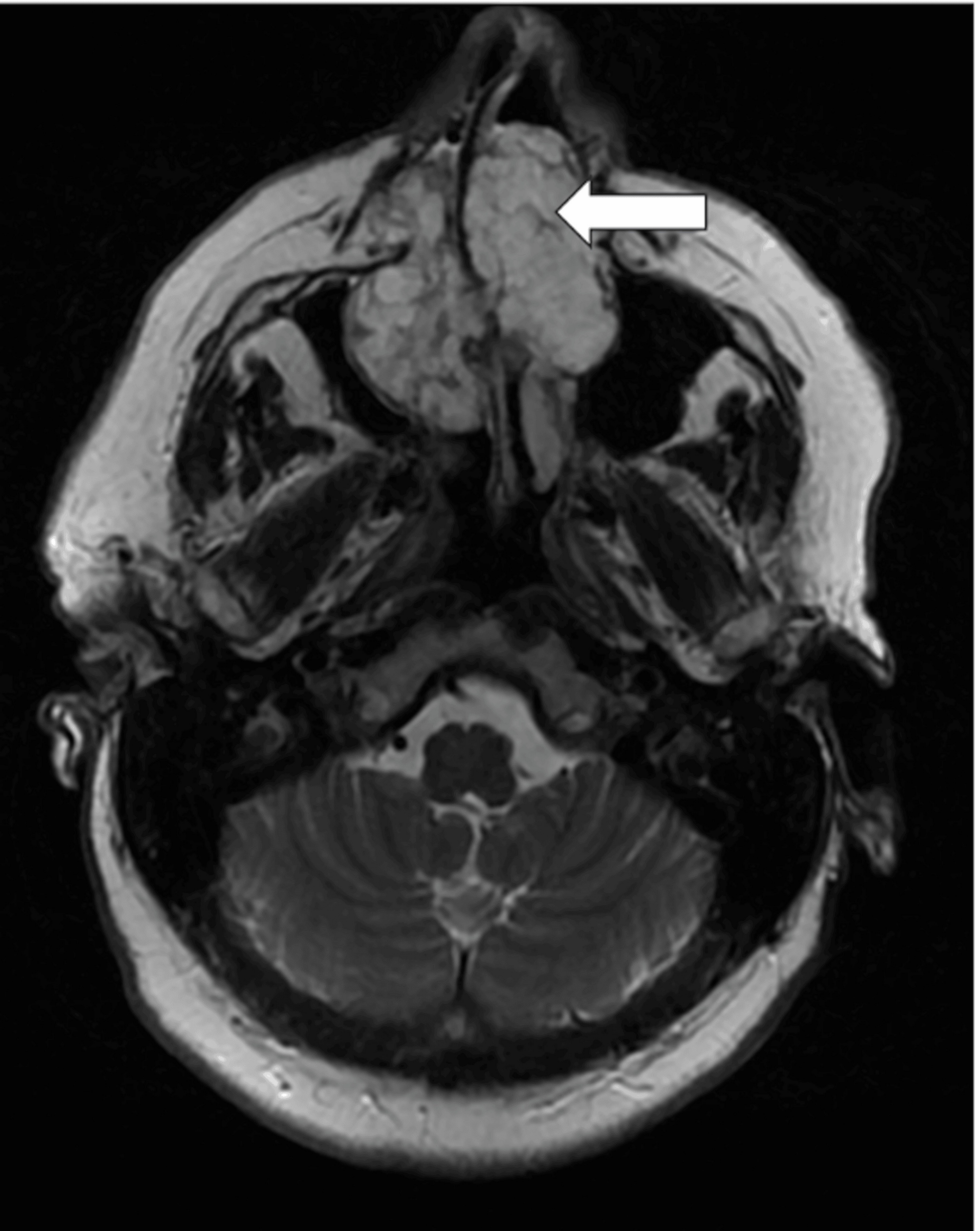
Axial T2-weighted MRI of sinonasal chondrosarcoma The image shows a lobulated mass centered on the anterior-inferior nasal septum, exhibiting marked hyperintensity with internal septations and focal areas of intermediate signal suggestive of chondroid matrix (white arrow). The lesion expands into both maxillary sinuses and the nasal floor, causing remodeling of adjacent structures while preserving the skull base MRI: magnetic resonance imaging
